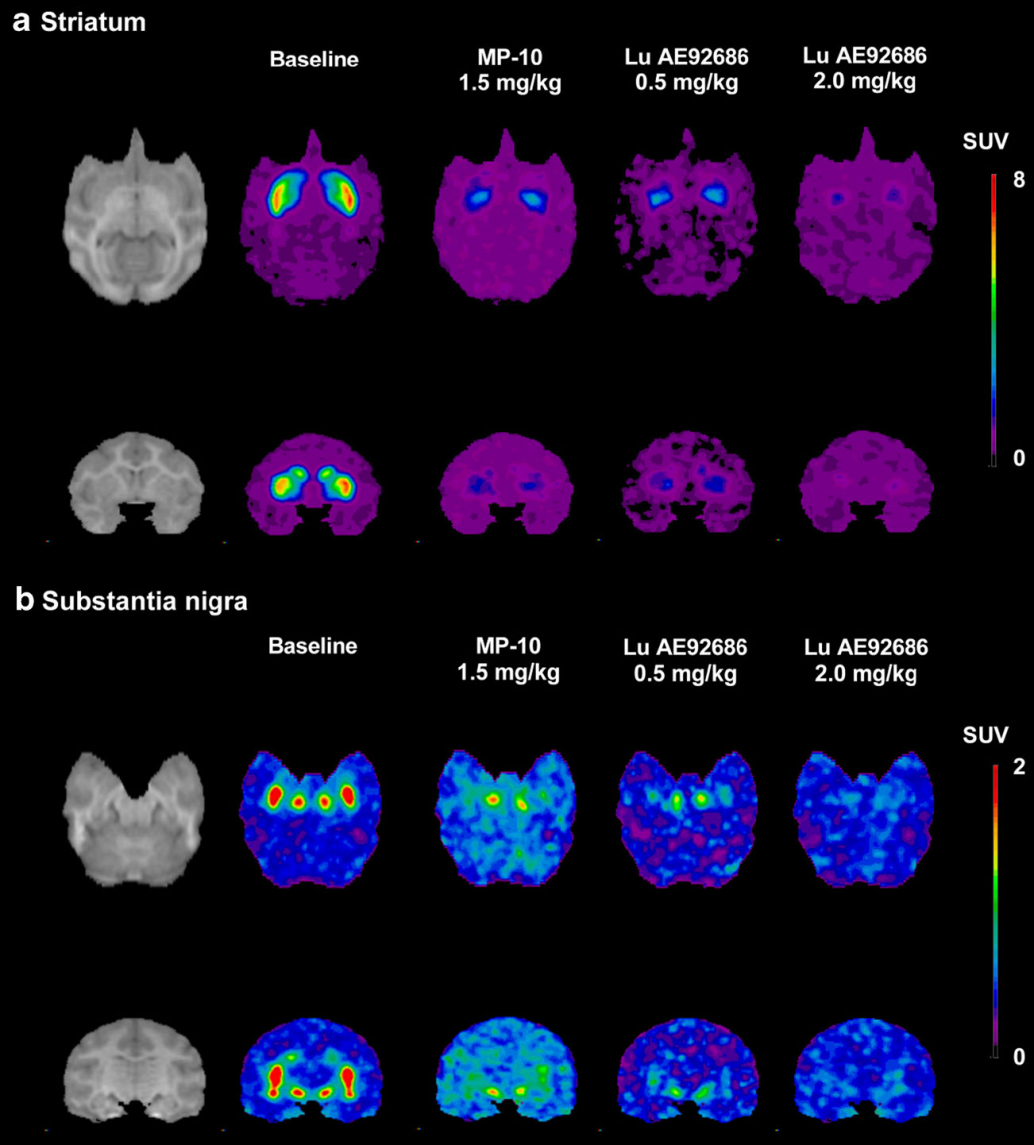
Magnetic resonance images and corresponding coregistrated PET summation images (average of frames from 9 to 123 min) of [^11^C]Lu AE92686 during baseline (mean of three baseline experiments) and three pretreatment conditions in NHP1. (a) Axial (top) and coronal (bottom) view of images at the level of striatum (b) Axial (top) and coronal (bottom) view of images at the level of substantia nigra; note the difference in the range of the standardized uptake value (SUV) colour bars between (a) and (b)
